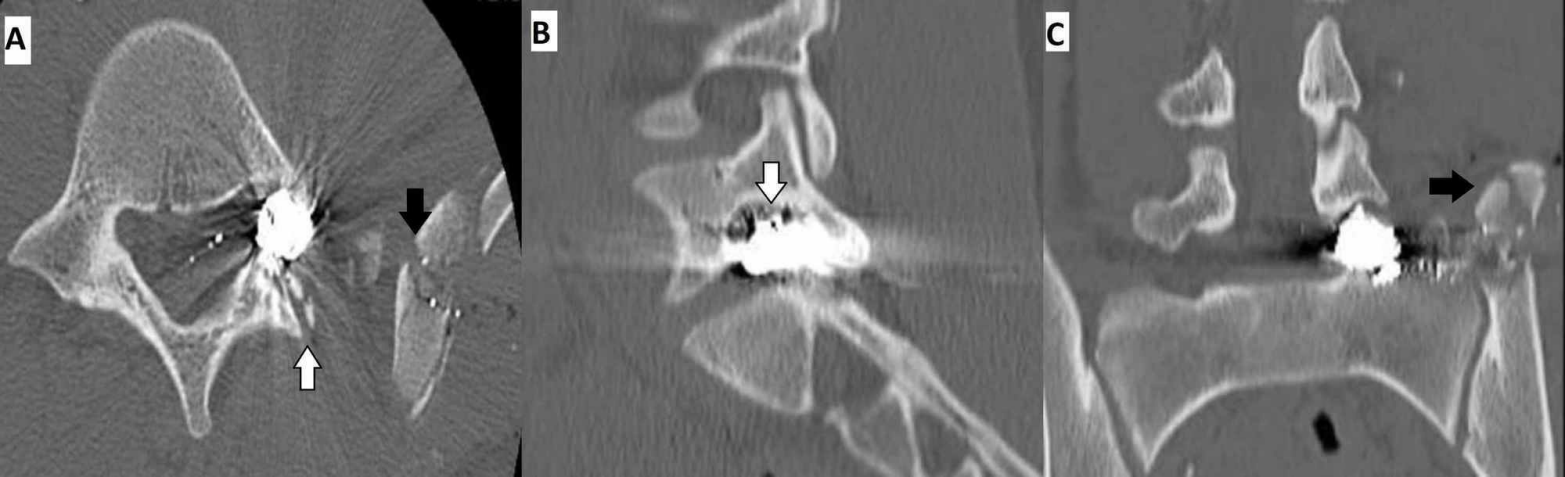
Preoperative CT scans demonstrating a high-density foreign body in the left articular facet of L5 neural foramen (A, B) axial and sagittal aspects showed fracture of the left L5 inferior facet (white arrows). No loss of vertebral body height or alignment. (A, C) axial and coronal aspects revealed comminuted blast fracture of the medial wing of the left iliac crest just superior to the left sacroiliac joint (black arrows), with no apparent joint destruction.
